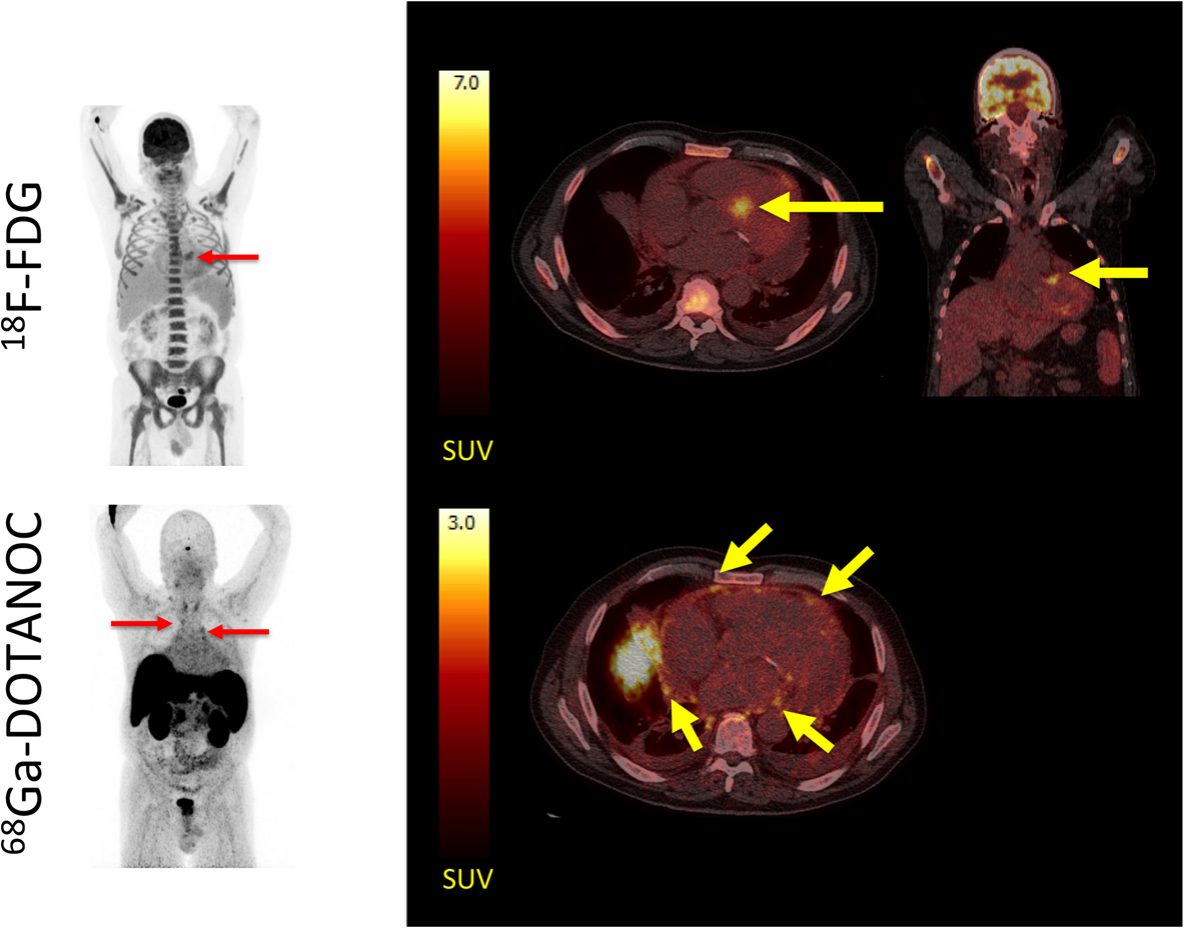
^18^F-FDG PET/CT false positive for cardiac sarcoidosis. *Left panel*: MIPs of patient no. 15 scanned after injection of ~370 MBq ^18^F-FDG and ~300 MBq ^68^Ga-DOTANOC. There was avid an ^18^F-FDG uptake in all areas of the bone marrow and the spleen indicating long-term infection. A few lymph nodes are visible in the upper mediastinum on the ^68^Ga-DOTANOC scan. *Right panel*: transaxial and coronal slices of the cardiac region revealed avid ^18^F-FDG uptake in the area around the aortic ostium (*yellow arrows*) which is often seen in aortic valve sclerosis, but can also be mistaken for uptake in a sarcoid lesion (SUVmax 7.6). Clear calcifications are seen around the posterior valve on the low-dose CT. On the ^68^Ga-DOTANOC scan (*bottom*), there is no activity above background in the myocardium. By contrast, activity is increased in the pericardium (*yellow arrows*) and some pericardial fluid is visible indicating pericarditis
